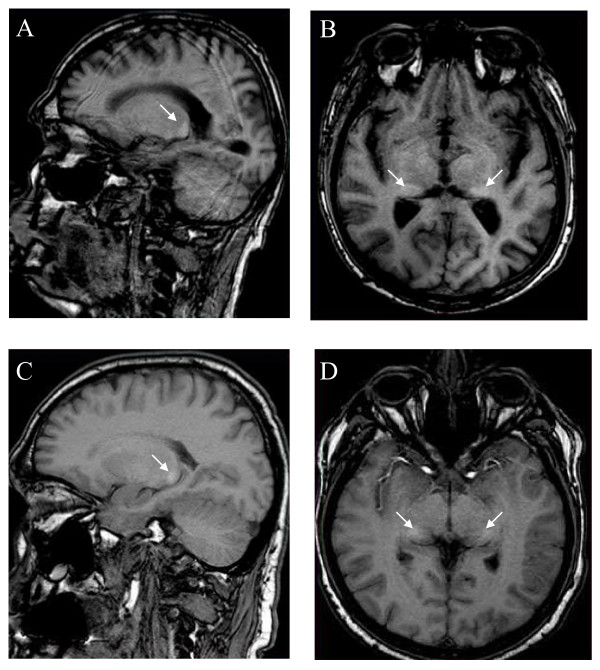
The pulvinar sign: T1-weighted sagital (A) and axial (B) MRI sections showing the pulvinar sign in a 66 year-old male patient. T1-weighted sagital (C) and axial (D) MRI section showing symmetrical high signals in the pulvinar region in a 42-year-old male patient. Courtesy: Dr Robert CARLIER and Dr Frédéric COLAS, CHU Raymond Poincaré, Garches, France.
